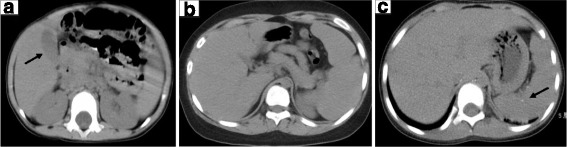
Abdominal CT scan. a Parenchymal low density lesions in the liver. b Lymphadenopathy in abdomen. c Calcification in spleen
